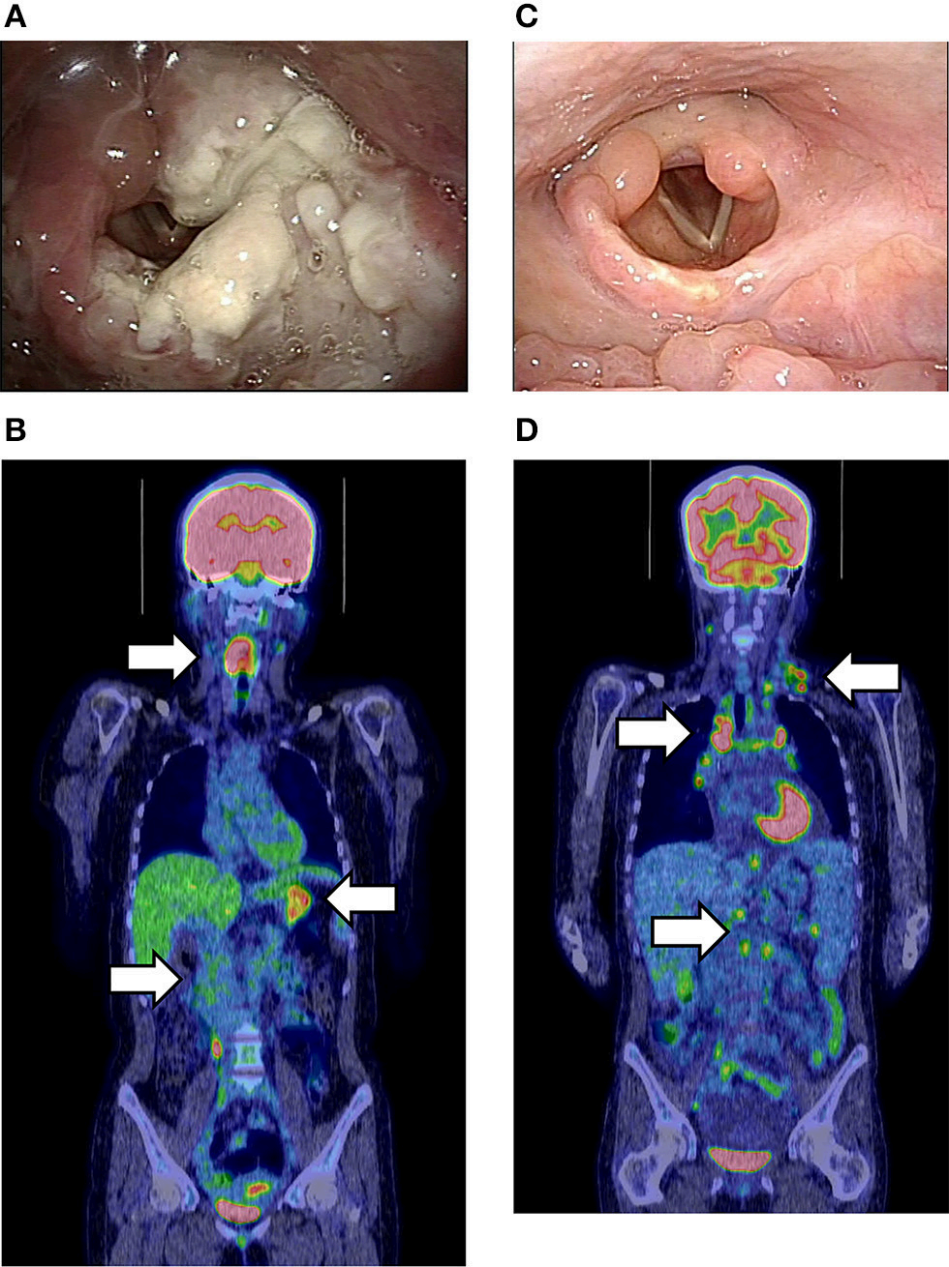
(A) Endoscopic findings of the laryngeal lesion of CD4^+^T-cell lymphoproliferative disease (LPD) lesion in case 4 prior to cancer chemotherapy. (B) Fluorodeoxyglucose-positron emission tomography (FDG-PET) at the onset of LPD. White arrows show the increased FDG uptake in the larynx, stomach, and terminal ileum. The maximum standardized uptake value (SUVmax) was 11.8. (C) Improvement of the laryngeal LPD lesion after bone marrow transplantation (BMT). (D) FDG-PET at the onset of donor-derived CD4+T-LPD after BMT. White arrows show the increased FDG uptake in multiple lymph nodes without laryngeal lesion. The SUVmax was 9.7.
